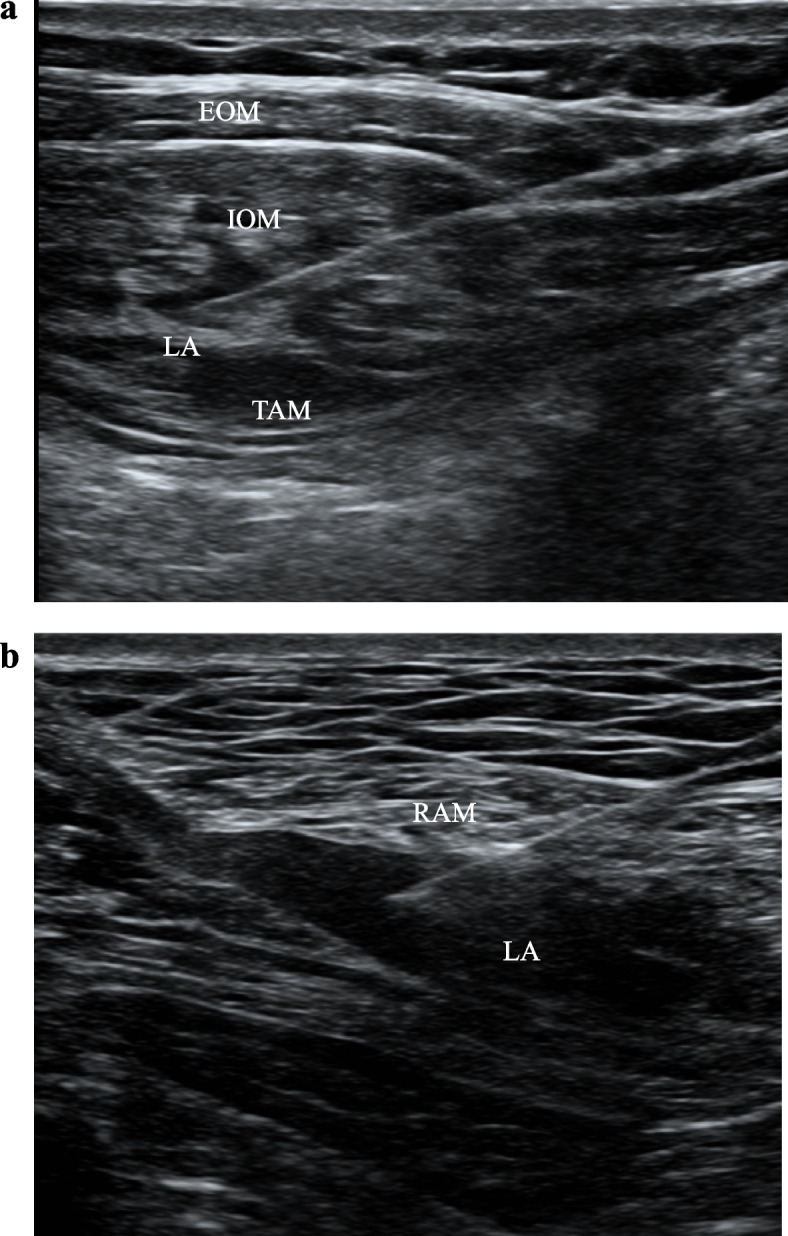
a Ultrasonographic image taken after the transversus abdominis plane block (TAPB). b Ultrasonographic image taken after the rectus sheath block (RSB). EOM – external oblique muscle; IOM – internal oblique muscle; TAM – transverse abdominal muscle; LA – local anesthetics; RAM – rectus abdominis muscle
